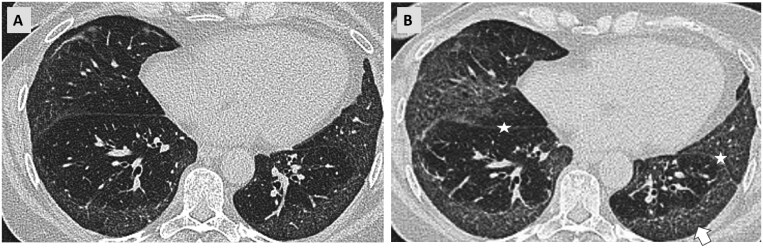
Progressive signs of fibrosis in a patient with Sjögren’s syndrome and pulmonary involvement showing an NSIP pattern. (A) Axial CT scan of the lower lobes from 2020 showing fine reticulations and ground-glass opacities with subpleural sparing. (B) Comparable axial section from a follow- up CT scan obtained in 2023 shows progressive fibrosis with progressive reticulations, emerging traction bronchiectasis (arrow) and progressive volume loss of the lower lobes as observed by the dorsal displacement of the fissures (stars).
